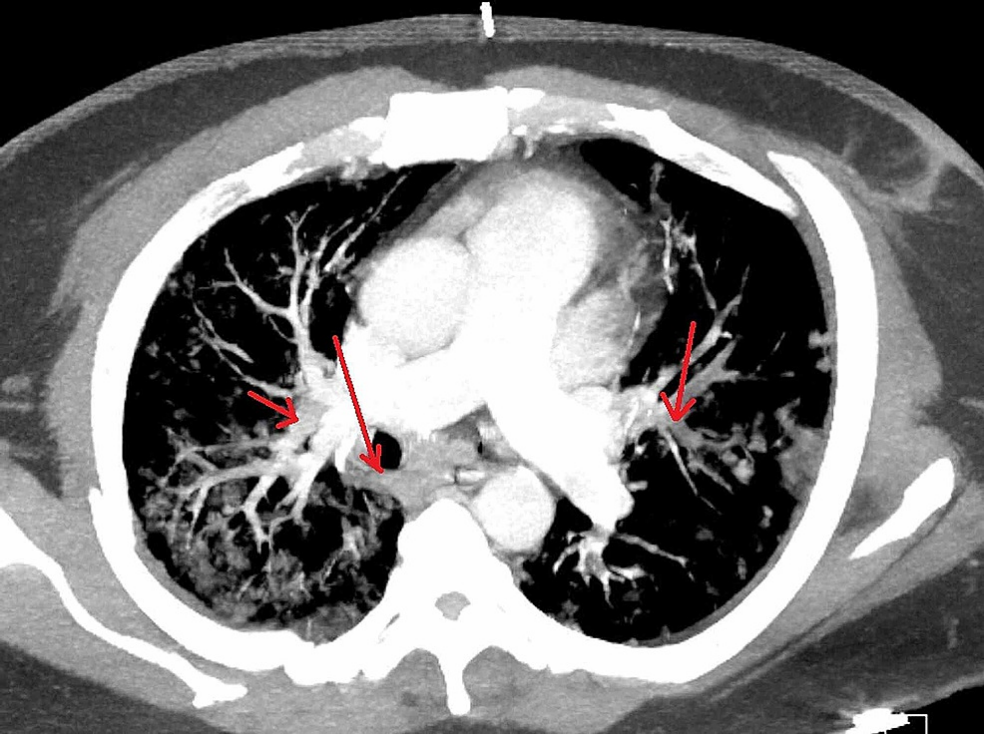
CT chest angiography showing ground-glass opacities and bilateral subsegmental pulmonary embolisms (red arrows).
